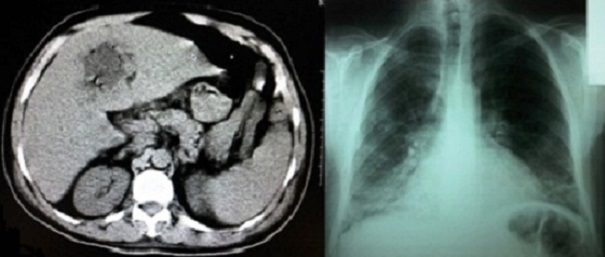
Tomodensitométrie abdominale avec injection de produit de contraste iodé, coupe axiale, montrant une masse hépatique de segment IV, radiographie pulmonaire de face montrant une image en faveur d'un foyer pulmonaire basal bilatéral
